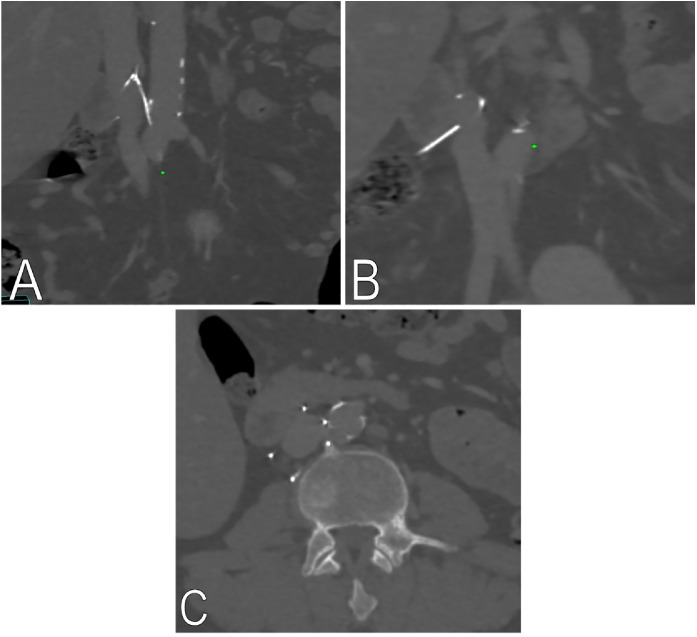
Multi-projection reconstruction images oriented (A and B) and axial CT reconstructions (C) demonstrating multi-directional IVC filter strut penetration. Two struts are adjacent to bowel loops, one strut is embedded in the distal abdominal aorta at the aortoiliac junction, and one fractured strut is projecting outside the right mesocolon.
